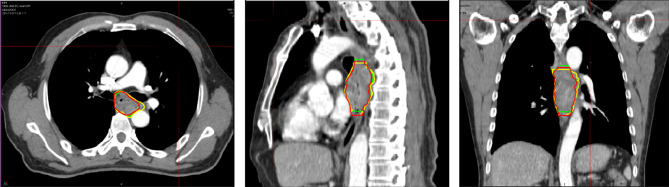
Representative images contoured in the image sessions in the transversal (a1), sagittal (a2), and coronal sections (a3) show differences among the three target volume delineations. The yellow contour represents the GTV_3D_, the red contour represents the GTV_PET-ref_, and the green contour represents the GTV_PET-reg._ GTV, gross target volume; GTV3D, GTV contoured based on 3DCT without referencing 18F-FDG PET/CT; GTVPET-ref, GTV contoured on 3DCT referencing 18F-FDG PET/CT; GTVPET-reg, GTV contoured on the deformed image derived from 3DCT and 18F-FDG PET/CT using MIM deformable registration software.
